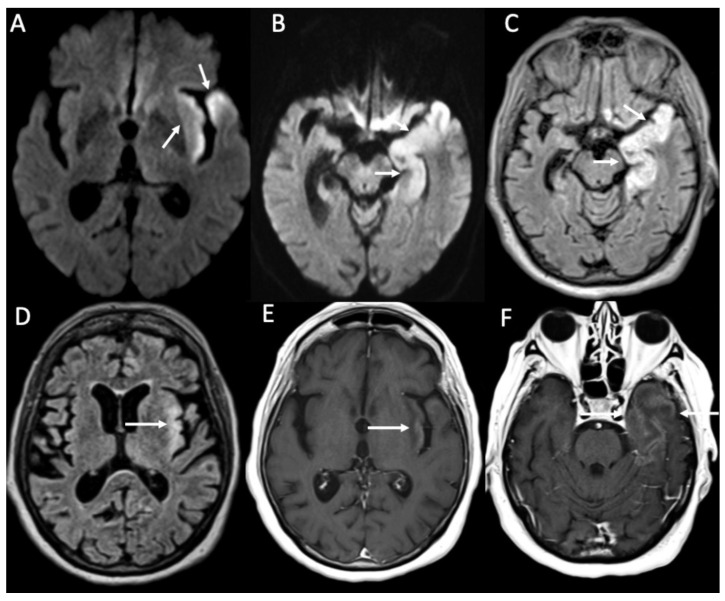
Axial DWI (A,B), FLAIR (C,D), and enhanced T1 (E,F) show fronto-temporo-insular signal alterations due to Herpes virus simplex type 1 encephalitis, which determines restricted diffusion (arrows in A,B), hyperintensity on FLAIR (arrows in C,D) and gyriform contrast enhancement (arrows in E,F).
